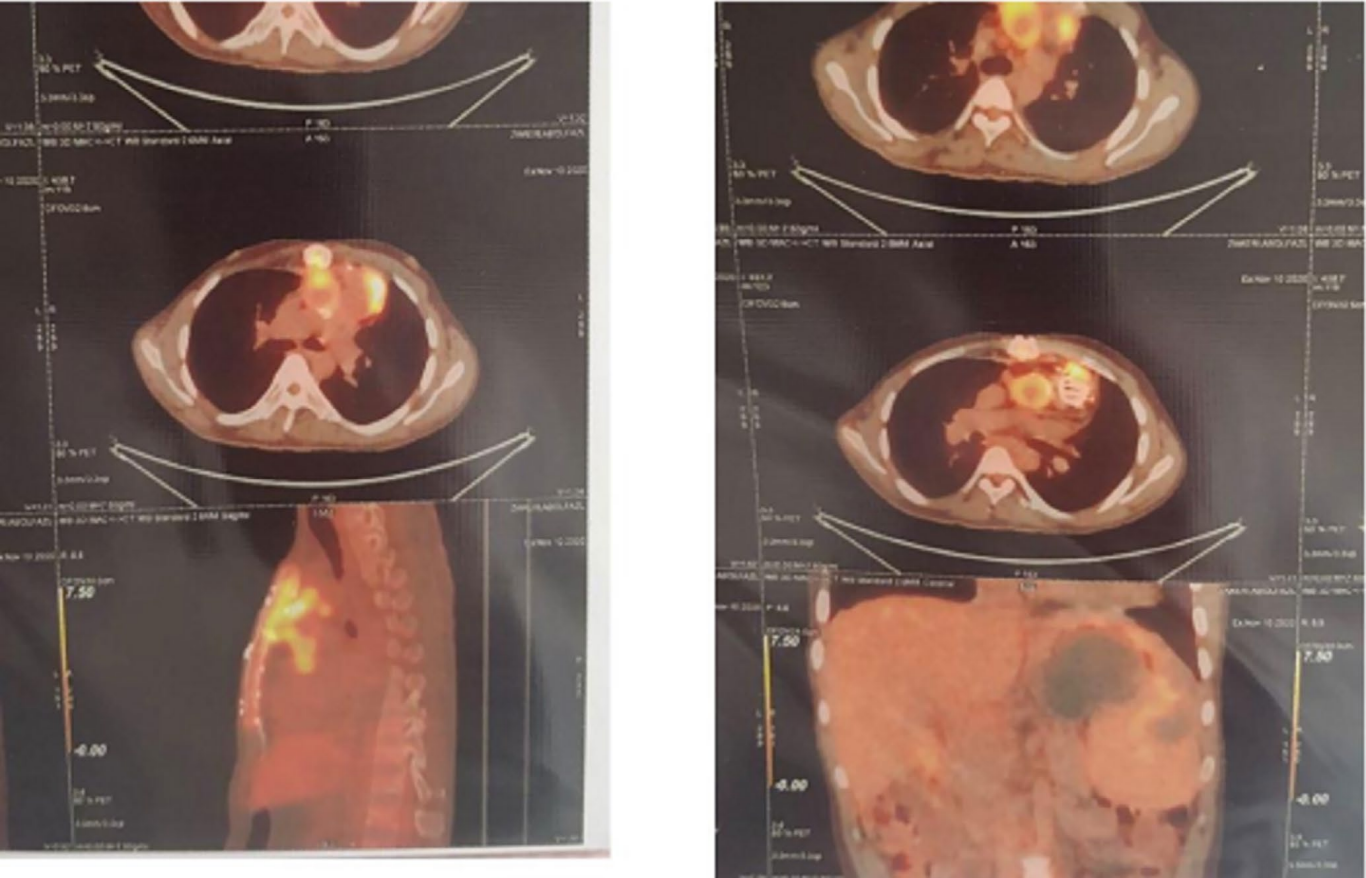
PET/CT scan: Hypermetabolic lesions around the aortic and pulmonary prosthetic valves, as well as involvement of the ascending aorta and proximal pulmonary artery.
